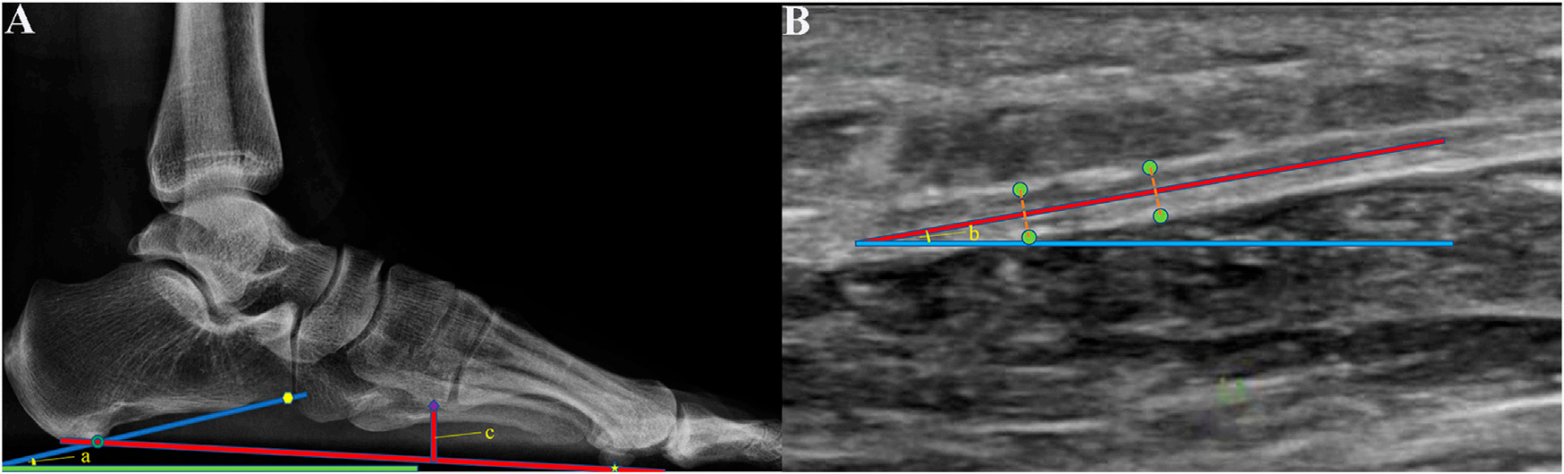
(A) Calcaneal pitch angle (angle a) and medial cuneiform height (c) were measured from X-radiographs of the weight-bearing foot. Calcaneal pitch angle is the angle between the supporting surface (green line) and the line (dark blue line) connected the lowest point of calcaneus (dark green circle) and the lowest point of the anterior edge of the calcaneus (yellow point). Medial cuneiform height is the distance from the lowest point of the medical cuneiform (the purple rhombic point) to the line that connected the lowest point of calcaneus (dark green circle) and the lowest point of the sesamoid under the first metatarsal (yellow five-point star). (B) Plantar fascia angle (angle b) was measured using B-mode ultrasound. It was defined as the angle between the middle line (red line) of the plantar fascia and the horizontal line (the blue line parallel to the probe and skin). The green points were the edge of the plantar fascia.
